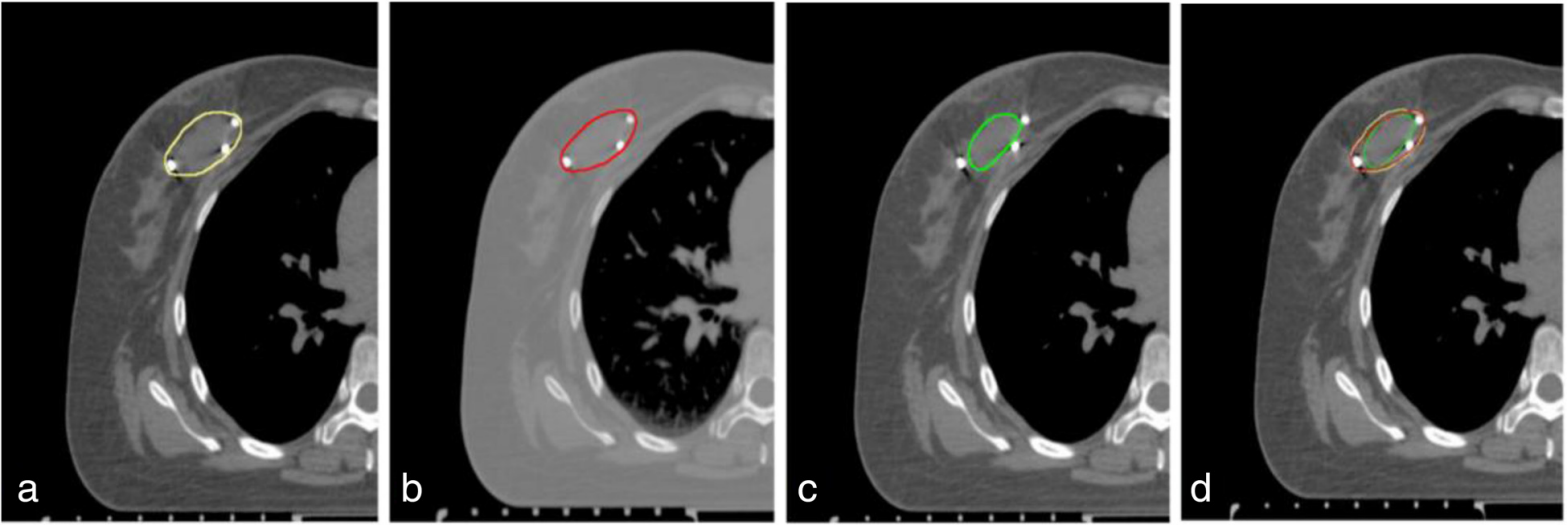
The outline of GTVc, GTVs and GTVc + s. a. GTVc + s: the gross target volume delineated based on both seroma and surgical clips; b. GTVc: clips the gross target volume delineated based on surgical clips; c. GTVs: the gross target volume delineated based on the seroma; d. GTVc + s (yellow), GTVc (red) and GTVs (green) on the same lay of a 4D-CT data set.
